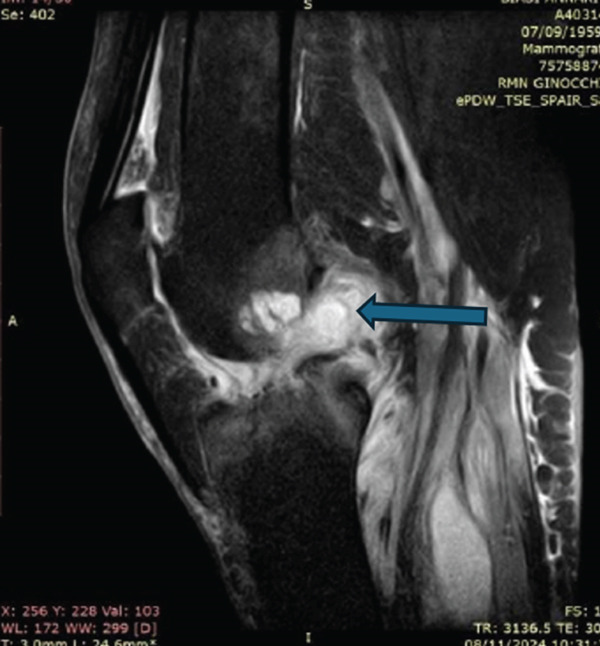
Computed tomography of the left knee, performed approximately Day 7. Annotated axial demonstrating joint effusion, intra‐articular gas, periarticular soft tissue oedema and early subcortical bone changes. Arrows indicate principal findings.
